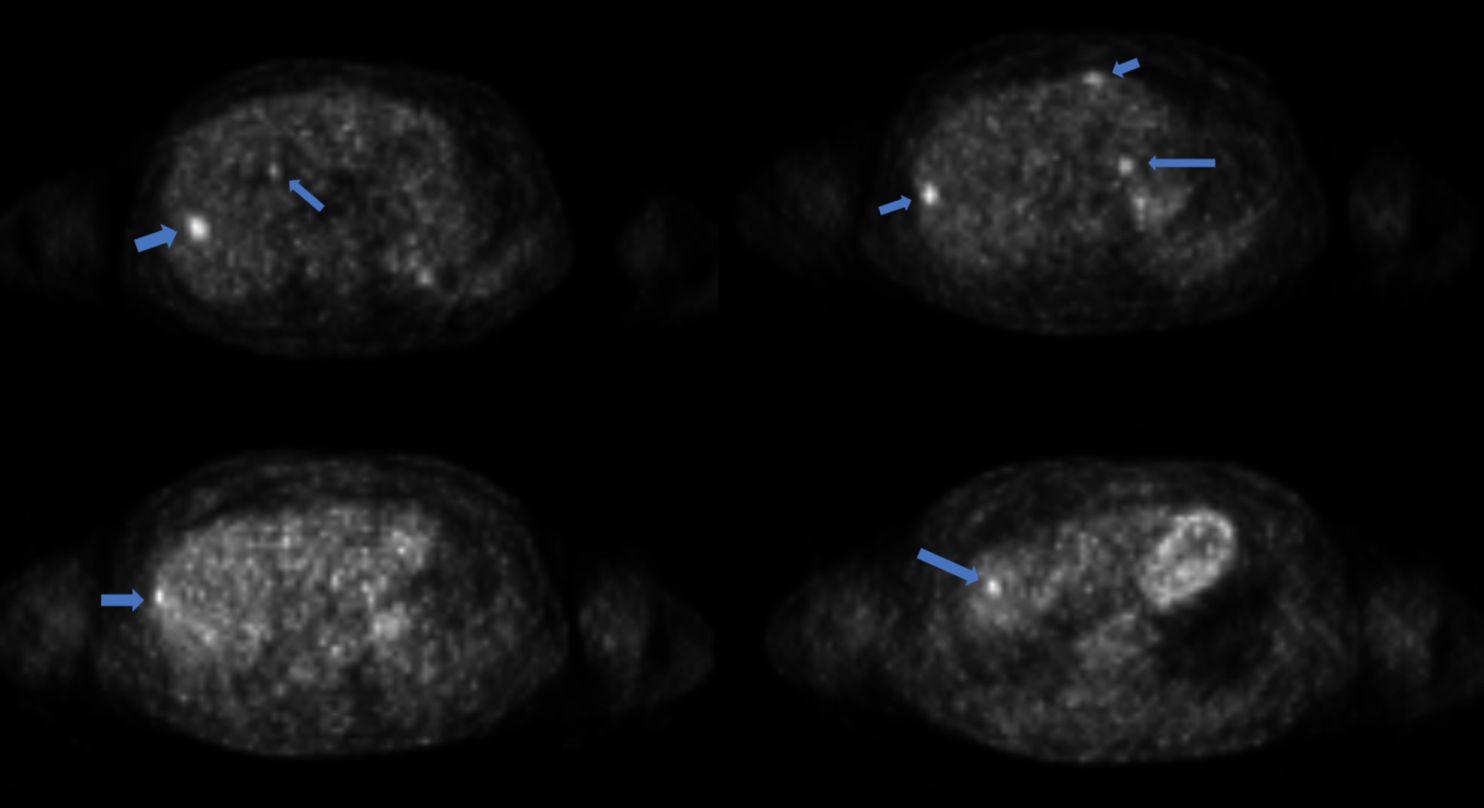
PET-CT scan of liver prior to radiation therapy PET-CT - positron emission tomography-computed tomography
